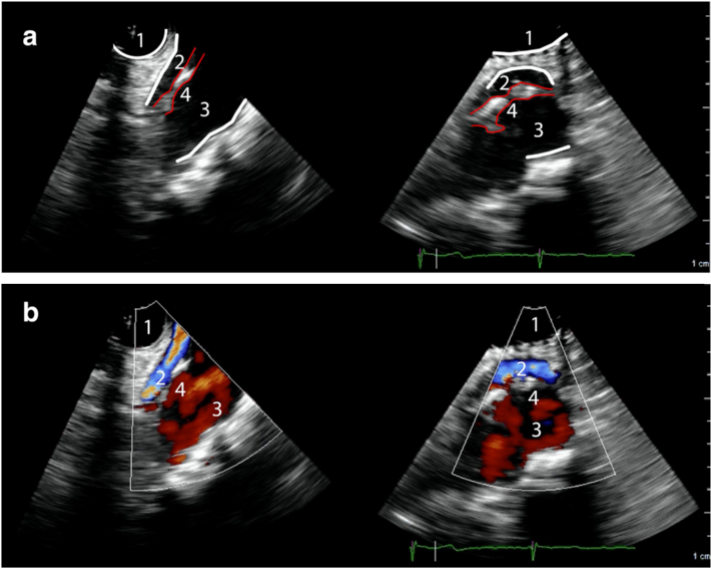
Panel (a) Modified TEE long- and short axis (X-plane) view of the distal ascending aorta (DAA), showing an intimal tear. Panel (b) Same image with color-Doppler showing differential flow in the true- and false lumen. 1 = Trachea with inflated endotracheal balloon; 2 = True lumen; 3 = False lumen; 4 = Intimal tear
